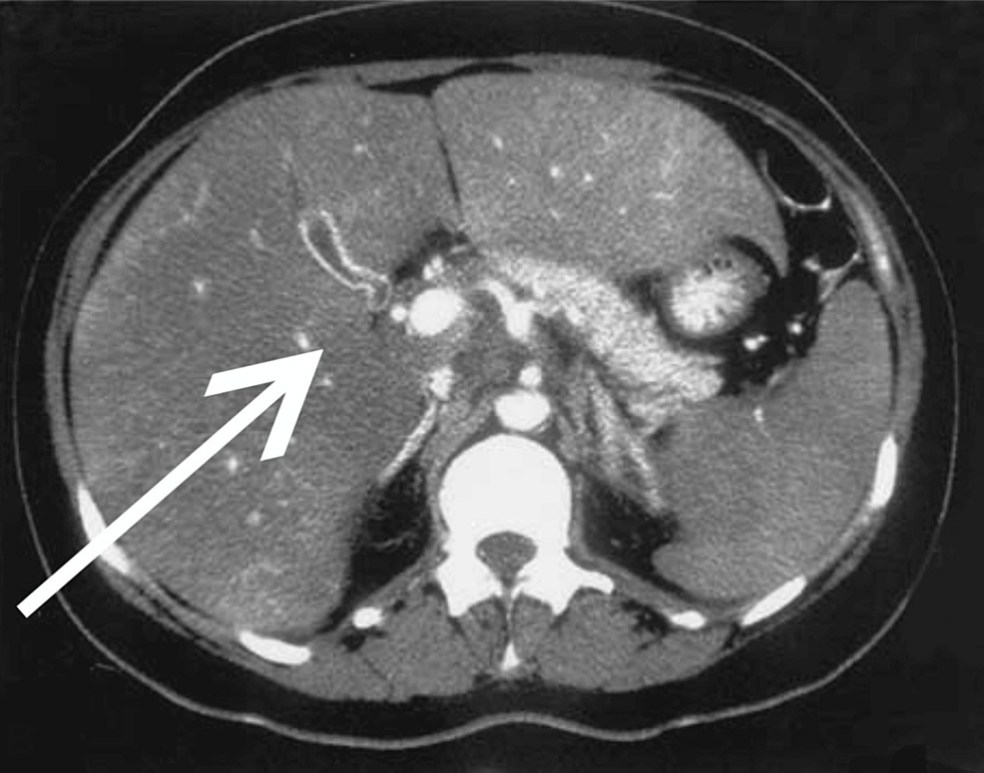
Abdominal CT showing diffuse hepatomegaly
